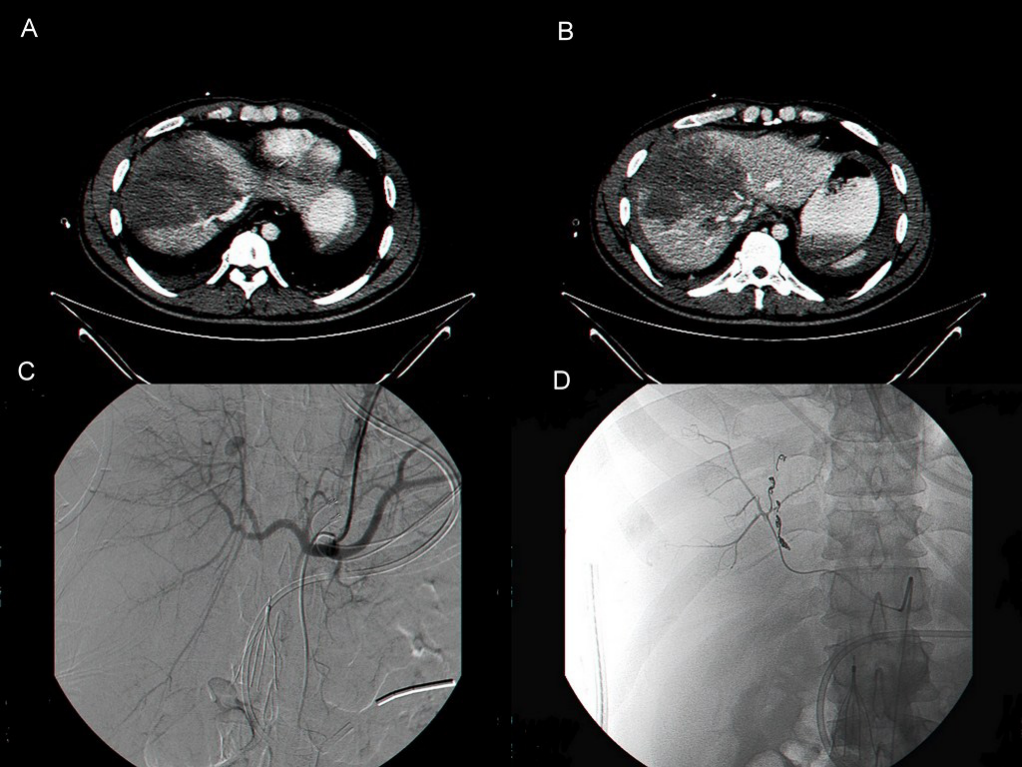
A and B - CT scan on admission showing grade IV liver trauma; C- Angiogram showing pseudoaneurysm on the right liver; D- Angiogram after embolization with coils.
